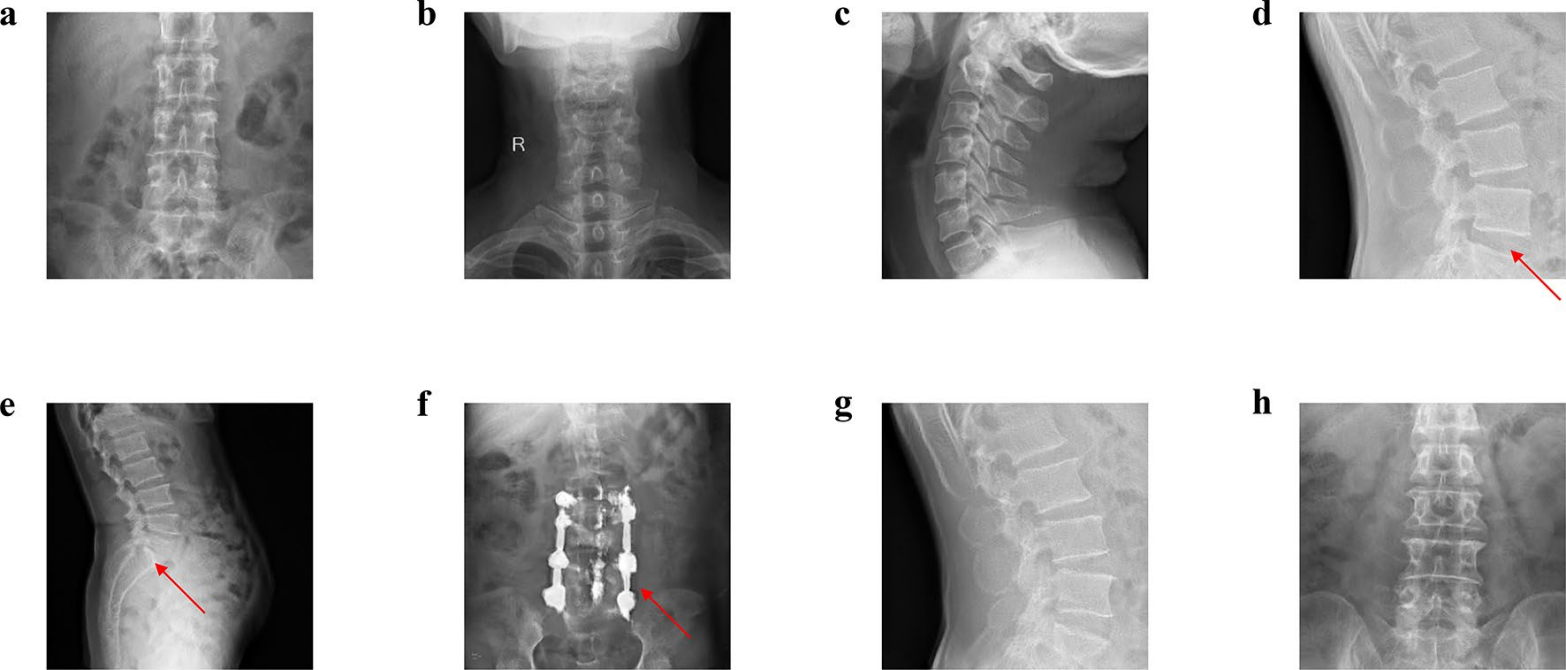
GAN generated synthetic spine radiographs specified to produce normal radiographs (a–d) and abnormal radiographs (e–h), (a–c, g–h) no finding, (d) disc space narrowing, (e) spondylolisthesis, (f) surgical implant.
